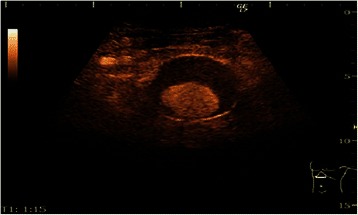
Abdominal contrast-enhanced ultrasound: abdominal aortic aneurysm.
